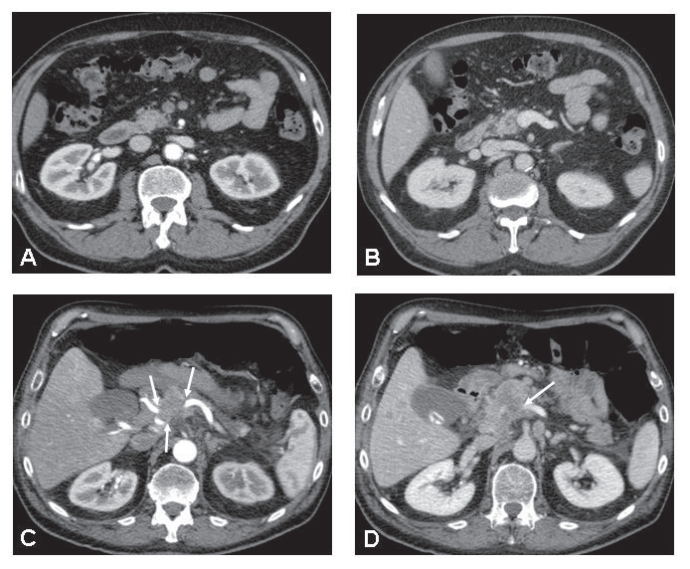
Contrast-enhanced CT (ceCT) shows a tumor-free superior mesenteric artery (sma) [A] and portal vein [B]. In another patient, ceCT suggests tumor infiltration of the celiac trunk [C] and the portal vein confluence [D] (arrows).
